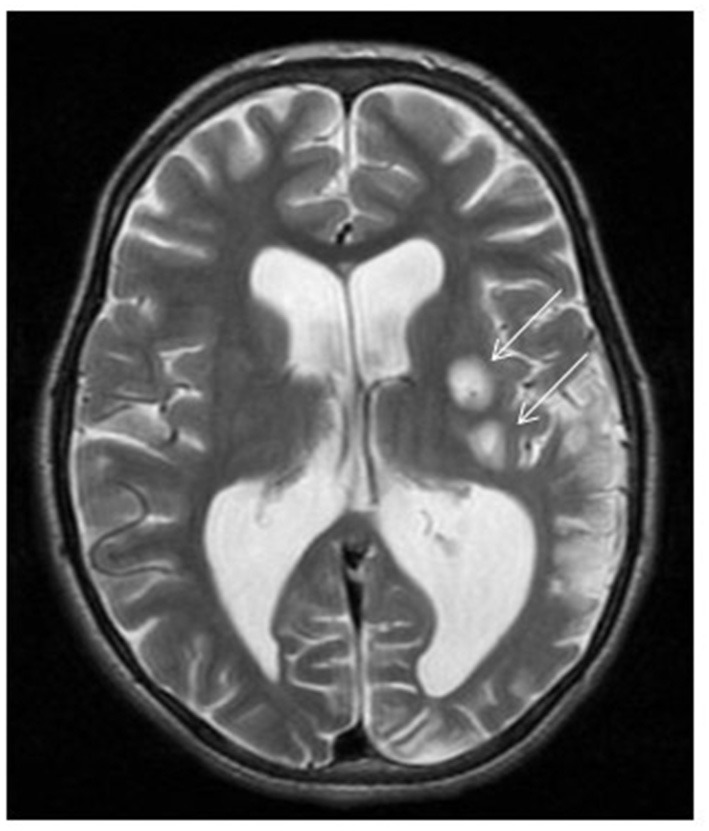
ND5*-related MELAS with stroke-like episodes. Brain MRI demonstrates two foci of abnormal high T2 signal intensity involving the left putamina (arrows) with restricted diffusion (not showed) representing acute insults. Left parieto-occipital area of encephalomalacia with paucity of the white matter. Mild ventriculomegaly more pronounced at left trigone due to ex vacuo dilatation.
